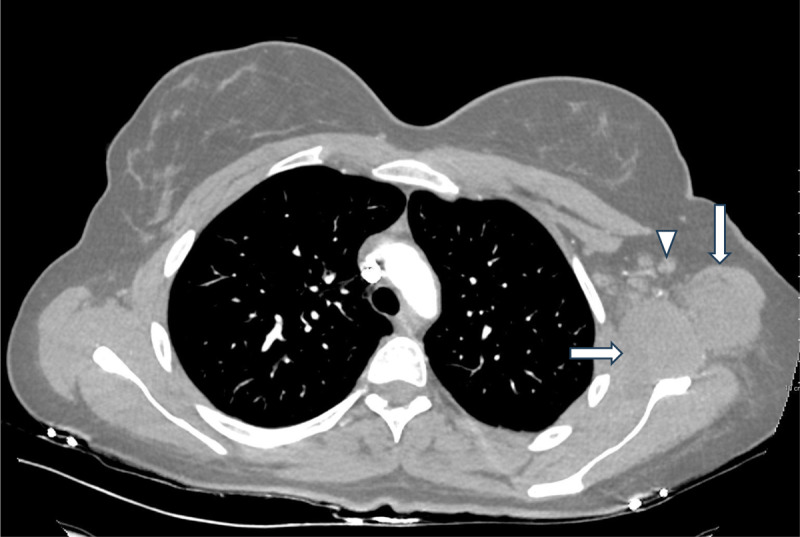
CT of the thorax showing swelling of the muscles of the left shoulder girdle (arrows) with surrounding fat infiltration and small adenopathy (arrow head) in the left axilla.
